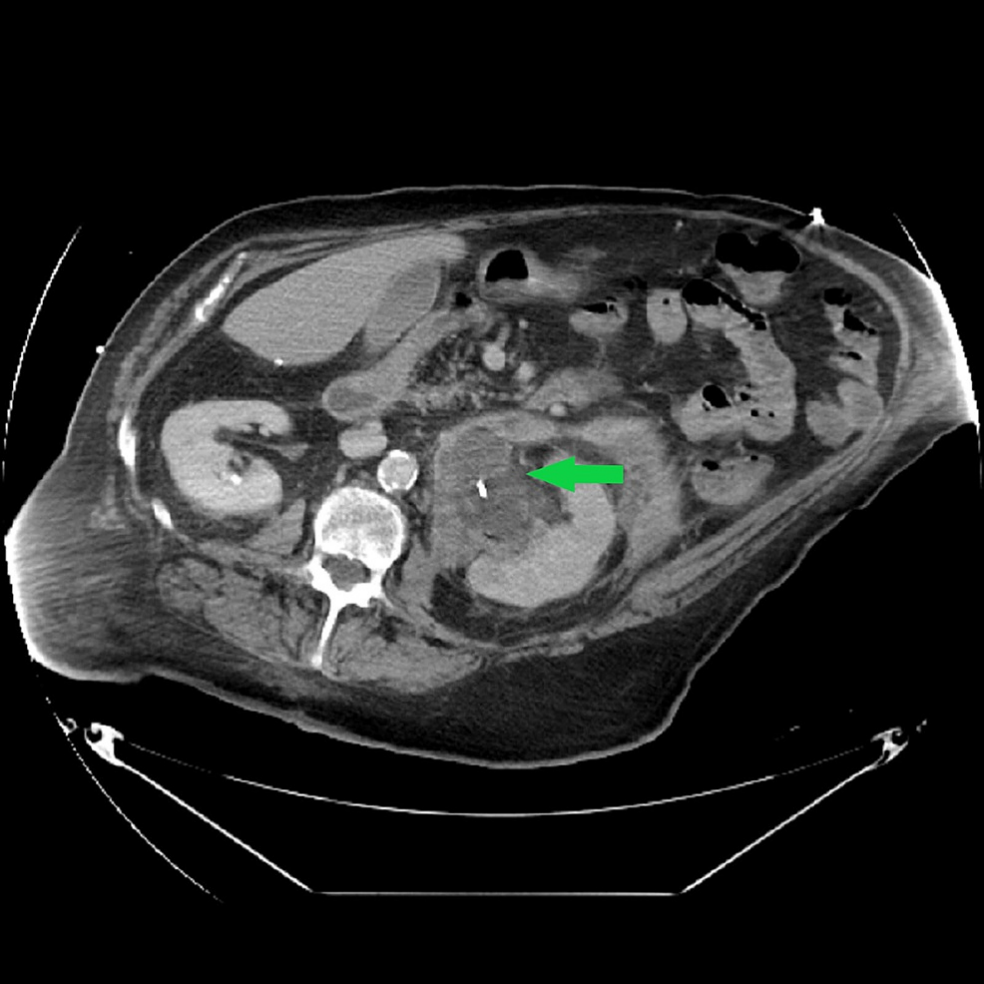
CT Abdomen and Pelvis Showing Renal Calculi at the Distal Left Ureter Causing Severe Left-sided Hydroureteronephrosis. There is a Multiloculated Fluid Collection in the Left Perinephric Space Representing Possible Perinephric Abscess.
